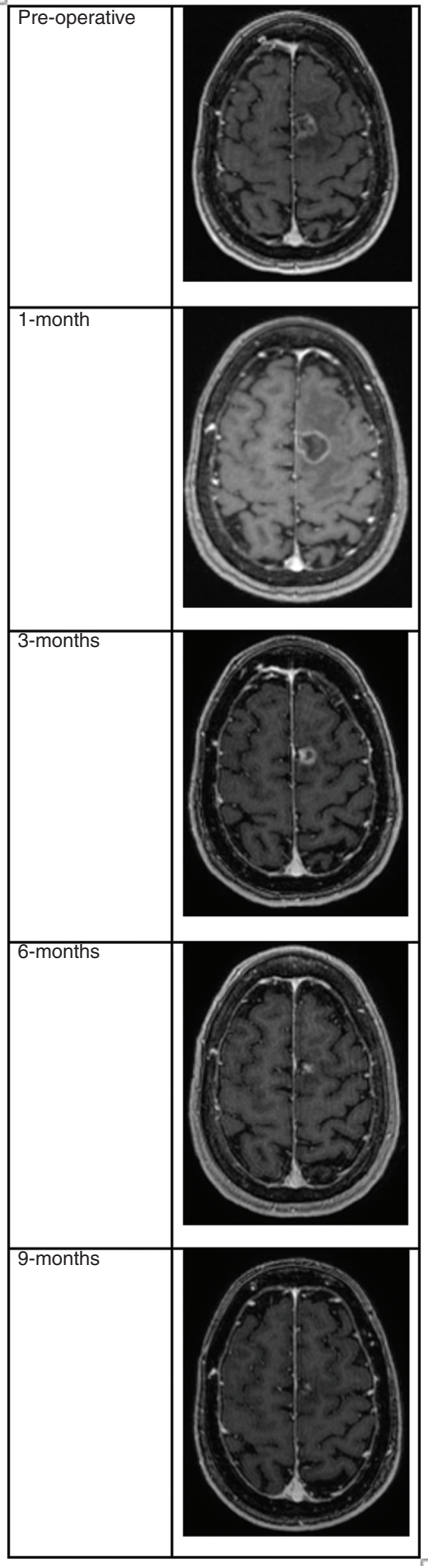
T1-weighted magnetic resonance imaging of a patient treated for recurrent metastatic lung cancer. Imaging progression shows typical evolution of effective laser interstitial thermal therapy (LITT) ablation with initial contrast-enhancing thin-rimmed expansion followed by contraction over the following months.
